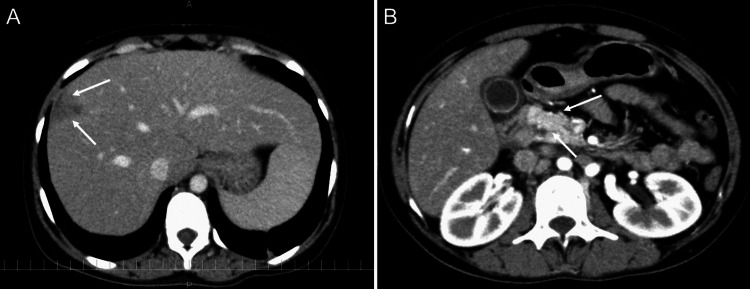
Abdominal enhanced CT scan image at the first hospitalization (A) Irregular mass shadows in the S8 region of the liver (white arrows) and (B) para-aortic lymphadenopathy (white arrows) were seen.
